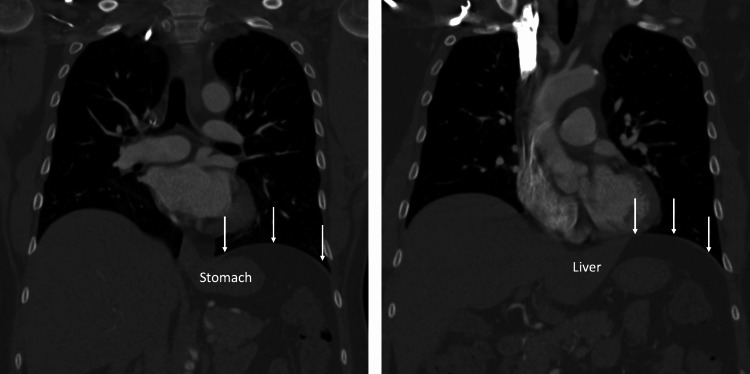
CT examples of the diaphragmatic region in the coronal plane noting the absence of pathology in this region. Arrows: diaphragm
